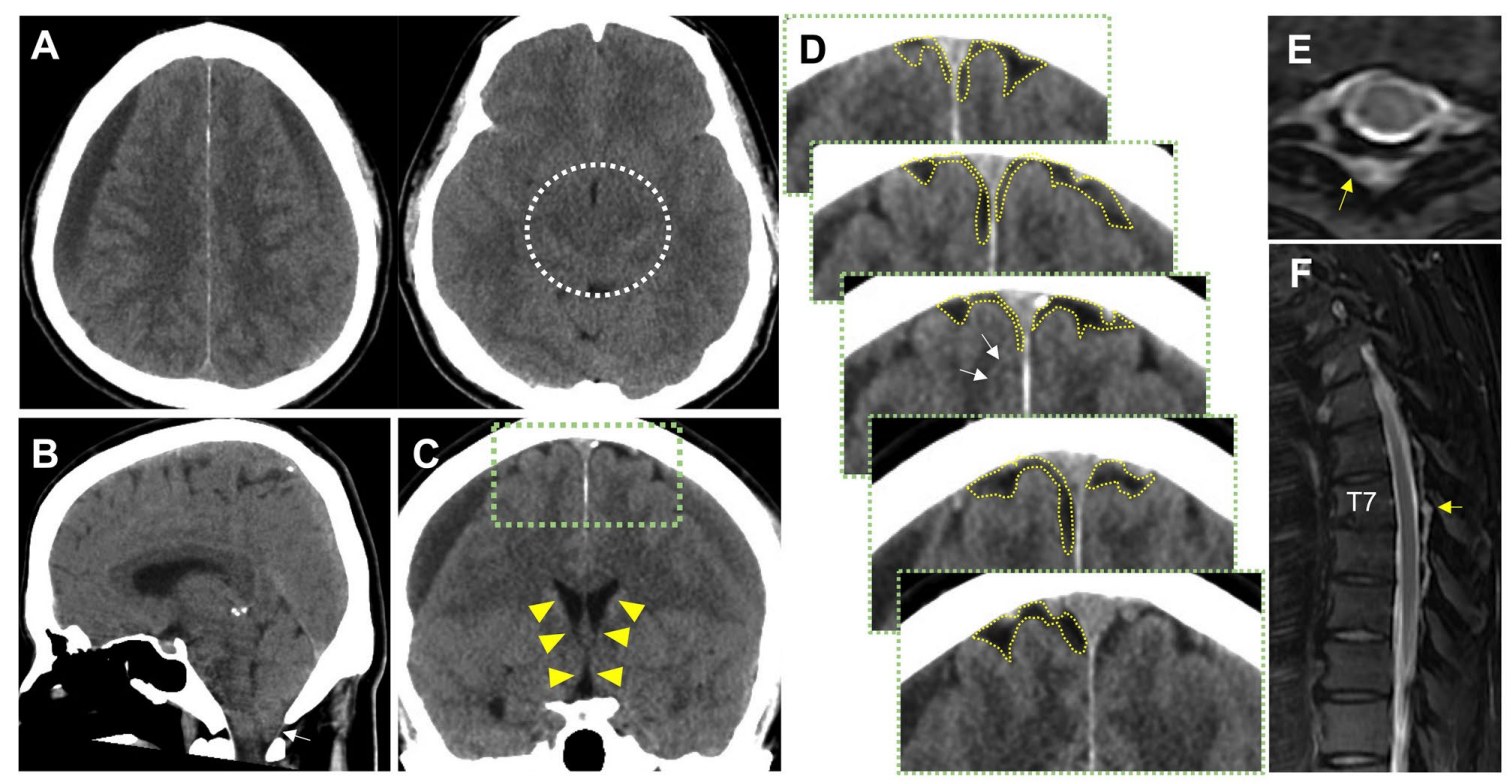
Initial CT findings of a case of bilateral CSDH with SIH (Case 8: male patient in his 50s, with headache but not orthostatic). Head CT showing (A: axial) (Left) symmetric bilateral CSDH, (Right) obliteration of crural-and-ambient cisterns (white dotted circle), (B: sagittal) cerebellar ptosis (white arrow), (C: coronal) slit-like ventricles (yellow arrowheads), (D) patency of cerebral sulci in the IHF (white arrows), and PSS (yellow dotted area) indicating possible SIH-induced bilateral CSDH. (E, F) T2-weighted image of spinal MRI showing high intensity in the epidural space at the level of T6-9, “CSF leakage (yellow arrow)”, proving SIH-induced CSDH
